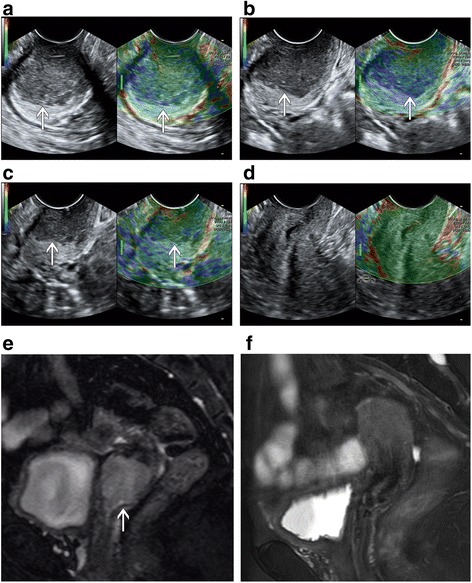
A patient with advanced cervical cancer (FIGO stage IIB) experienced complete response to concurrent chemo-radiotherapy(CCRT). B-mode and elastography images show a significant decrease in strain ratio in cervical cancer (arrow): 4.17 prior to CCRT a 3.03 at week 1 during CCRT b 2.73 at week 2 during CCRT c and 1.4 post CCRT d Corresponding axial T2-weighted images exhibited a significant decrease in the maximal diameter of tumor (arrows): 3.5 cm at pre-therapy e and 0 cm post therapy f
